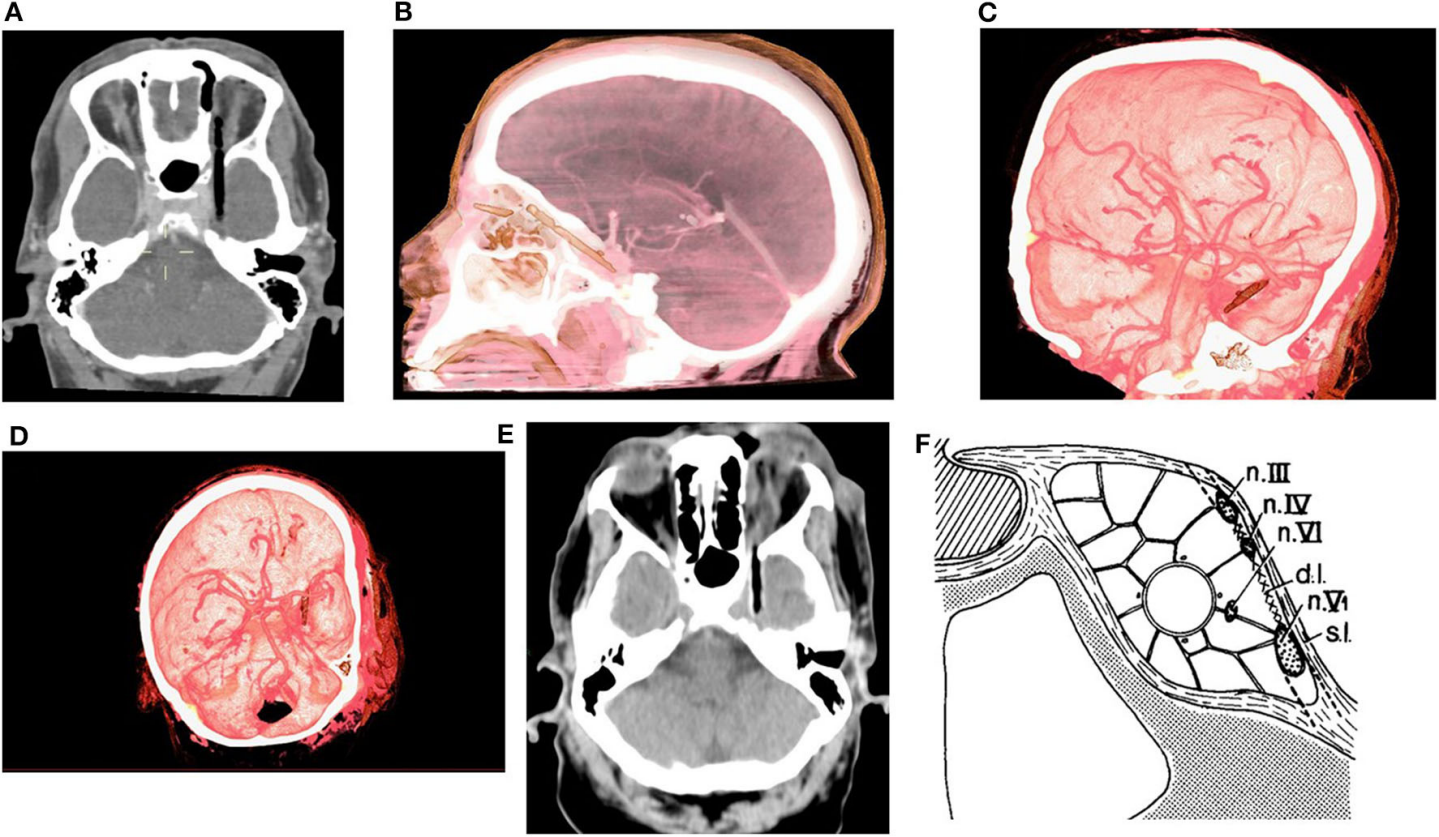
(A) Pre-op CT scan axial view, (B) Pre-op CTA 3D reconstruction lateral view, (C,D) Pre-op CTA 3D reconstruction oblique views. (E) Axial view from CT scan which was performed after the orbital stage of the surgery. (F) Lateral wall of CS [s.l, superficial layer; d.l, deep layer; Modified from (18), with permission].
